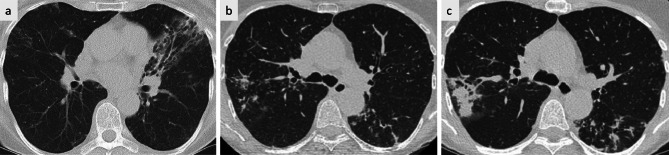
Thoracic CT scans (axial plane, 1-mm-slices). a: CT thorax scan 12/2018 revealing bronchiectasis predominantly in the left lung (Lingula). b: Nodules and consolidations consistent with progressive NTM pulmonary disease in 12/2020. c: Disease progression under macrolide-based antimicrobial regimen in 01/2022
